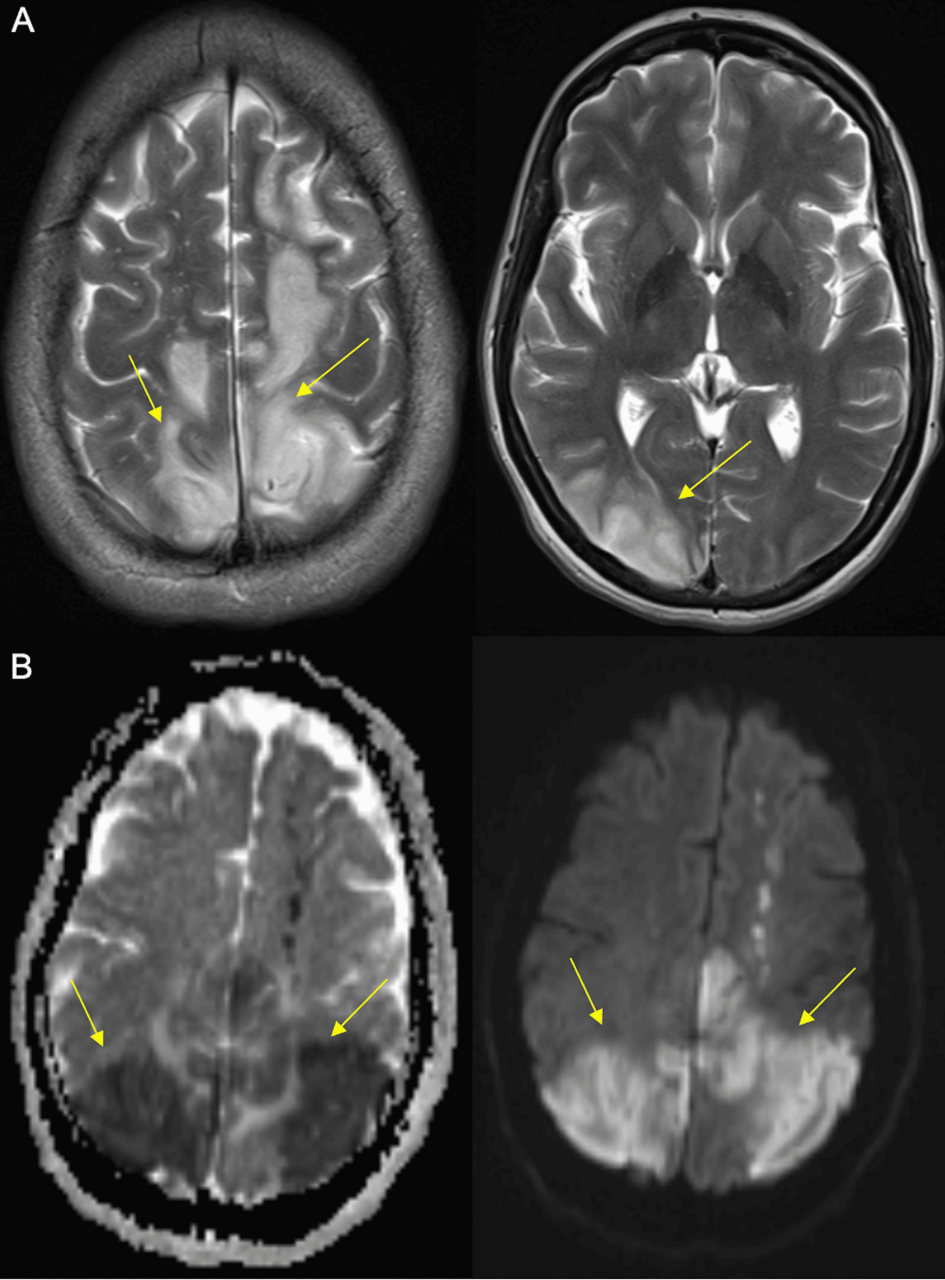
Brain MRI at admission Panel A: axial T2-weighted images. Panel B: axial ADC image on the left and diffusion-weighted image on the right side (yellow arrows pointing to areas of cytotoxic edema) ADC: apparent diffusion coefficient; MRI: magnetic resonance imaging
